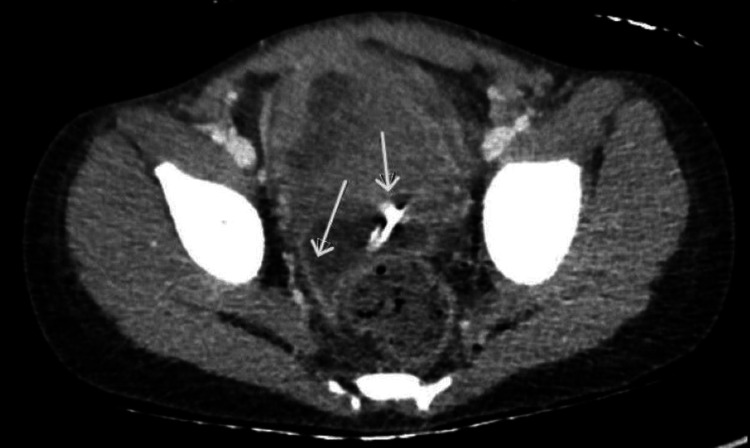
CT abdomen and pelvis (right arrow) showing PD catheter with enhancement of the nearby peritoneal wall (left arrow) CT: computed tomography; PD: peritoneal dialysis
